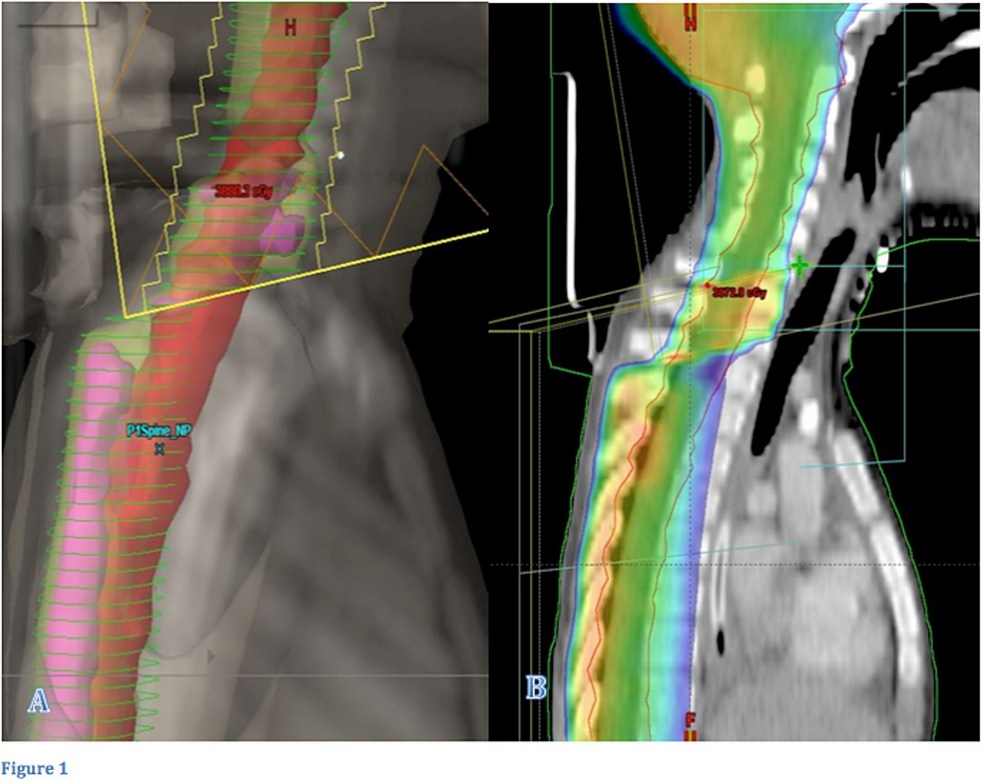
Comparison of two plans of a medulloblastoma child patient with and without field-in-field technique and modulated junctions A: Sagittal DRR of a medulloblastoma child planned without field-in-field technique. Note the junction of the craniocervical field with the PA field showing deficient coverage of the anterior area of the PTV with 95% coverage (shown in green lines) of the prescribed radiation dose and the subsequent hot spot areas showed with purple-colored dose distribution areas. B: Sagittal CT images of the same patient planned with field-in-field technique and modulated junctions, and dose coverage is showed in color wash. The junction can now be seen covered with ≥95% coverage of the prescribed dose with minimum hot spot areas. DRR, Digitally reconstructed radiograph; PA, posteroanterior; PTV, planning target volume.
